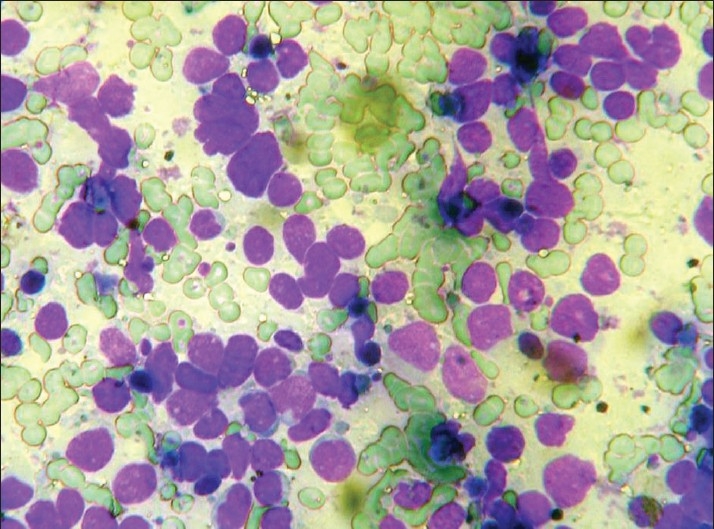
Small cell carcinoma of lung: CT-guided FNAC smears (MGG, ×400)
